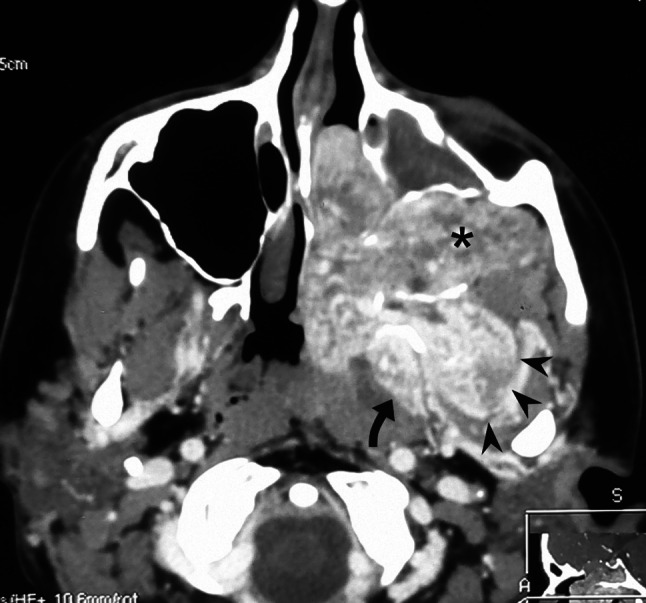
Contrast-enhanced axial CT scan well demonstrates three routes of tumor lateral spread in a case of extensive JNA: into the pterygopalatine and infratemporal fossa (*asterisk*), posterior to the pterygoid process (*curved arrow*) and between its plates (*arrowheads*)
